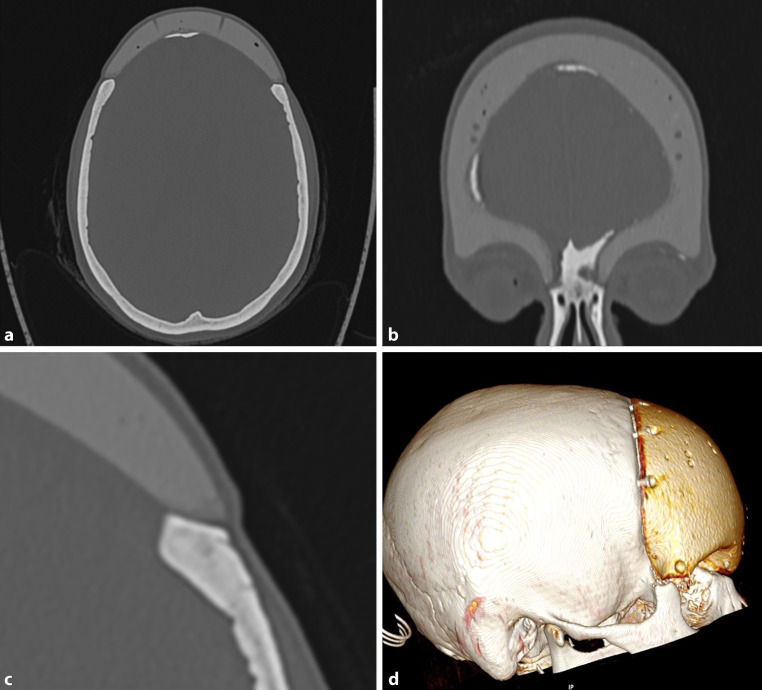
CT scans of a carbon fiber-reinforced resin implant after resection of a fibrous dysplasia of the frontal bone, 22 years after implantation. a This scans show an Axial view, the calcification of adjacent dura, bore holes in the implant for suturing the dura to the implant. b Same patient in a Coronal view. c The axial view shows sufficient implant-bone contact. d 3‑D reconstructions shows the positioning of the screws
